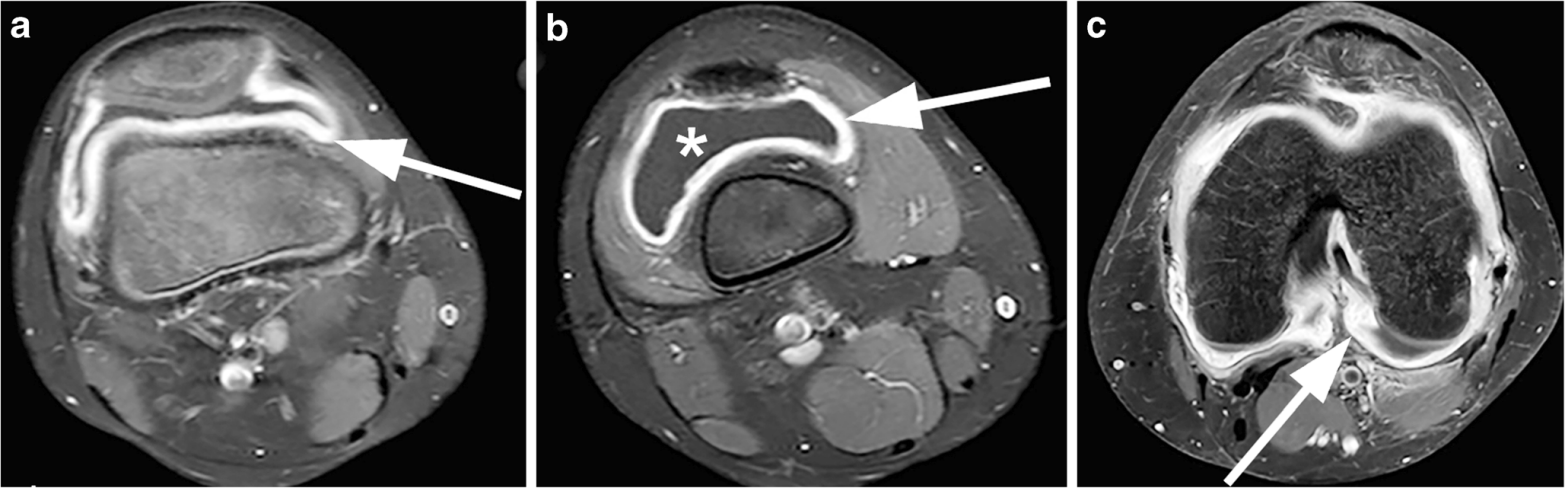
Axial T1-weighted MRI of the knee with fat suppression after gadolinium-based contrast administration in three patients with juvenile idiopathic arthritis shows synovial hypertrophy in the central locations of the knee. a Enhancing synovial hypertrophy in the retropatellar region (arrow) in an 9-year old girl. b Enhancing synovial hypertrophy in the suprapatellar region (arrow) in an 11-year old boy. Notice the non-enhancing low-signal-intensity joint fluid in thesuprapatellar recess (*). c Enhancing synovial hypertrophy around the cruciate ligaments (arrow) in a 15-year old girl
